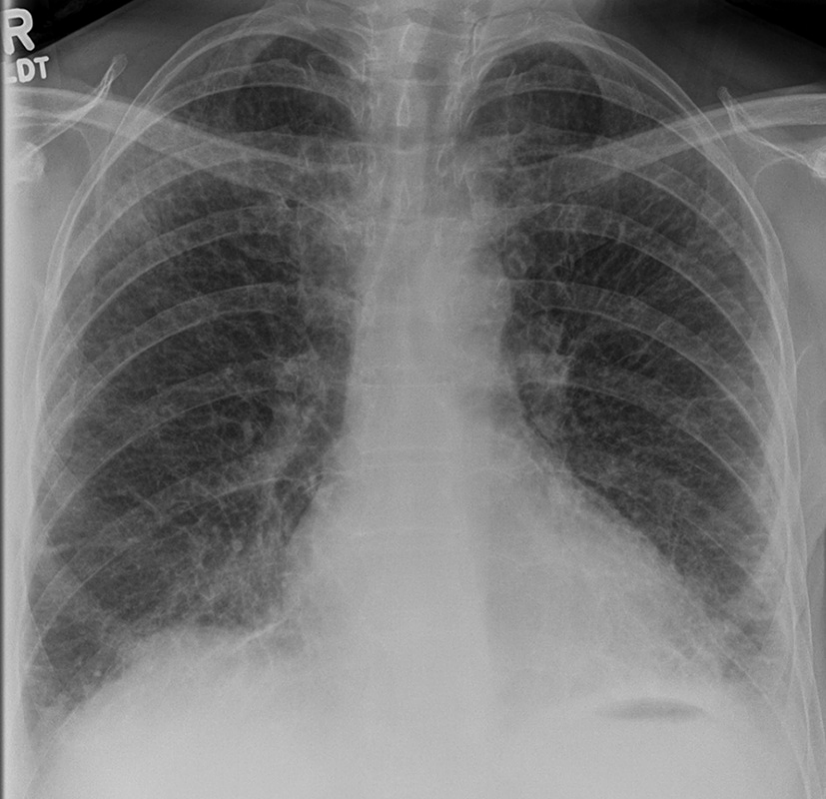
Chest radiograph. Diffuse, abnormally thickened interstitial septal lines and small bilateral pleural effusions.
